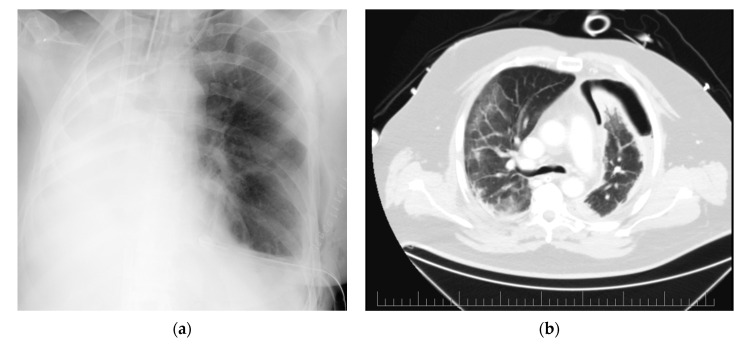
Radiographic images of presented cases: (a) Postoperative chest X-ray of a patient after left-sided double atypical resection and previous right-sided pneumonectomy (ECMO cannulas introduced via right internal jugular and femoral veins); (b) Preoperative CT scan of a patient with bilateral COVID-19 pneumonia with a ground glass and crazy paving pattern and left-sided pneumothorax.
